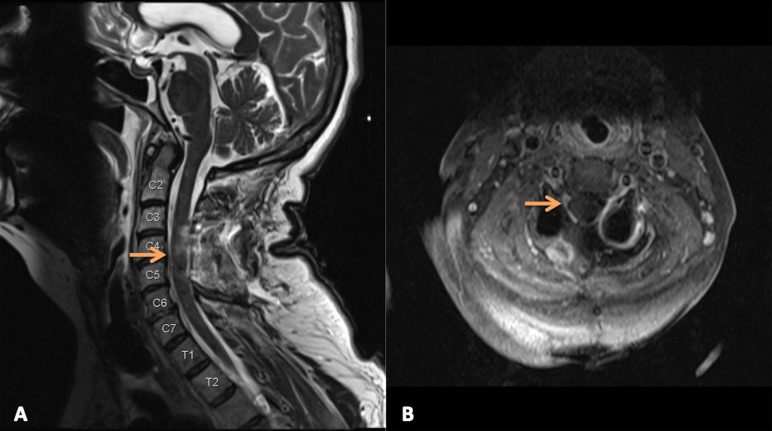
Postoperative MR Imaging A) Postoperative T2 sagittal axial MRI shows gross total resection and decompression of cord B) Postoperative T2 axial MRI shows gross total resection and decompression of cord
